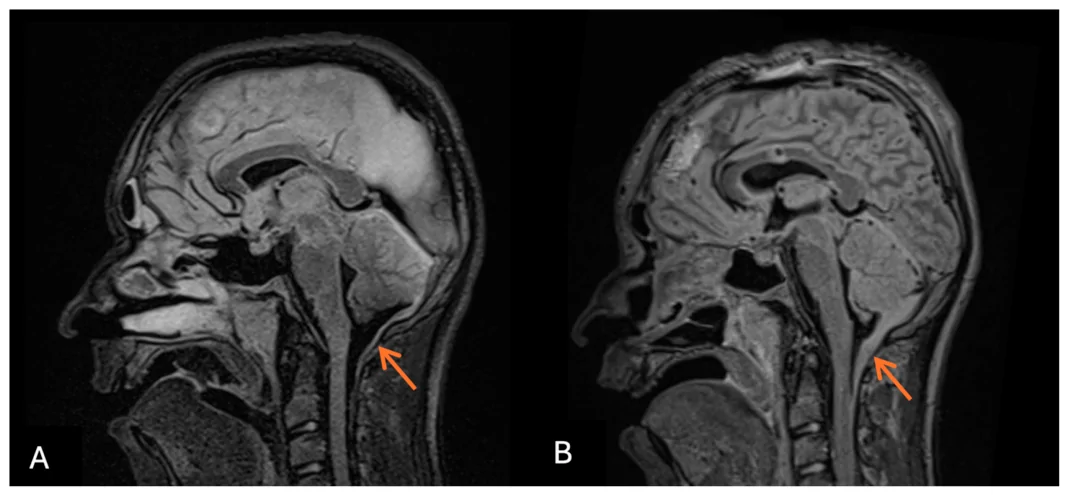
Sagittal FLAIR MRI on hospital day 1 (A) and hospital day 3 (B) demonstrating marked infratentorial progression of the empyema. The arrows in (A,B) show the progression of the extra-axial empyema measuring up to 7.7 mm extended along the midline and dorsally into the spinal canal.
